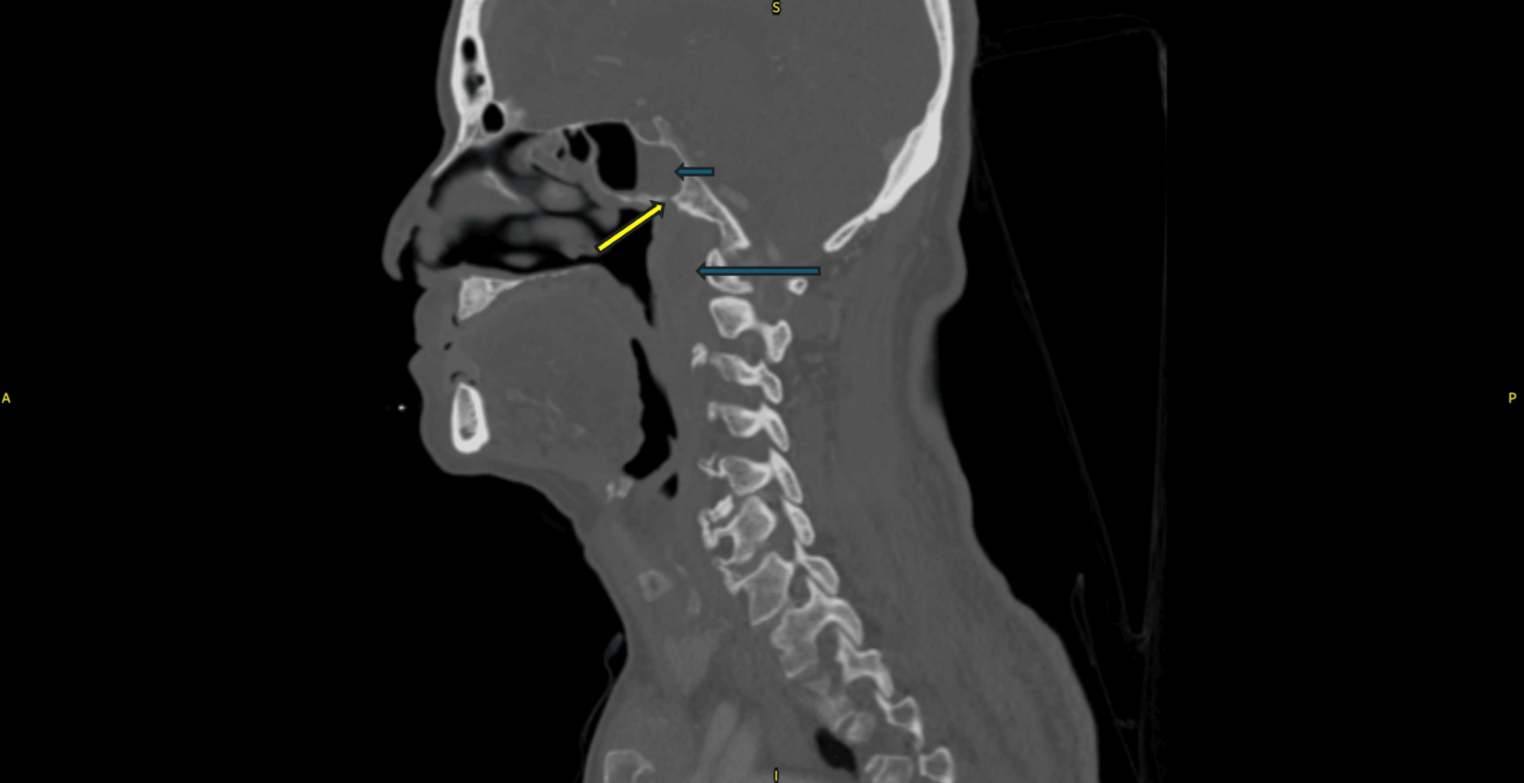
Contrast-enhanced CT (bone window) scan in sagittal view Isolated sphenoid collection in the sphenoid sinus (short arrow), thickened prevertebral space at the nasopharyngeal region (long arrow), and bony lytic erosion at the floor of the sphenoid sinus (yellow arrow) are observed.
